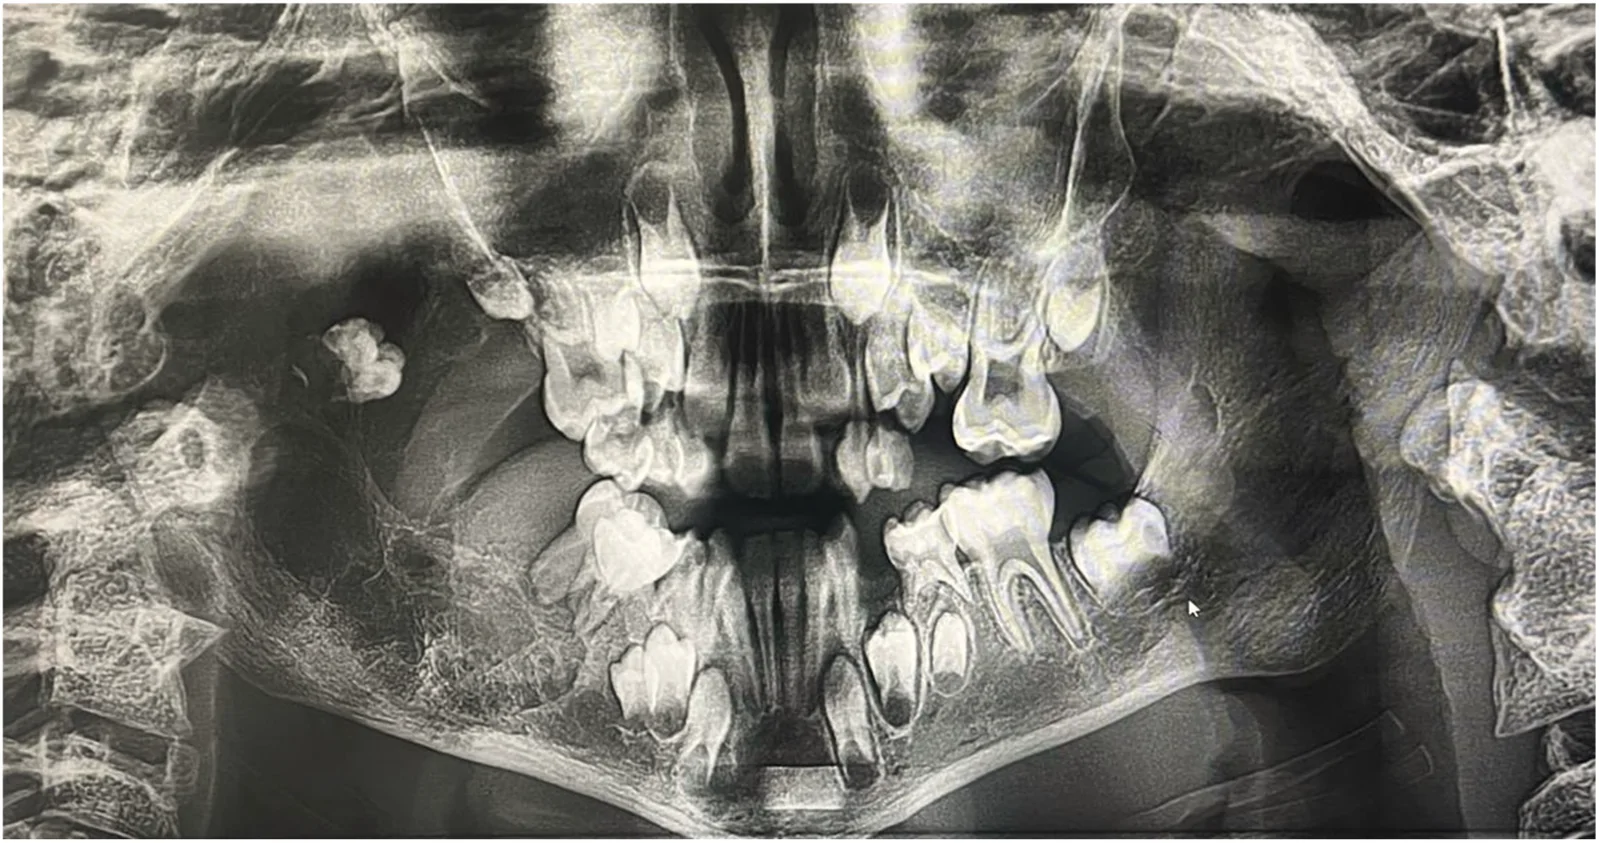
Panoramic radiograph showing: progression of the metastatic lesion in the region of the mesial root of tooth 46, with persistent arrest of root development. displacement of the tooth germs of teeth 44 and 45. Persistent displacement of tooth 47 toward the mandibular ramus, with ongoing coronal development.
